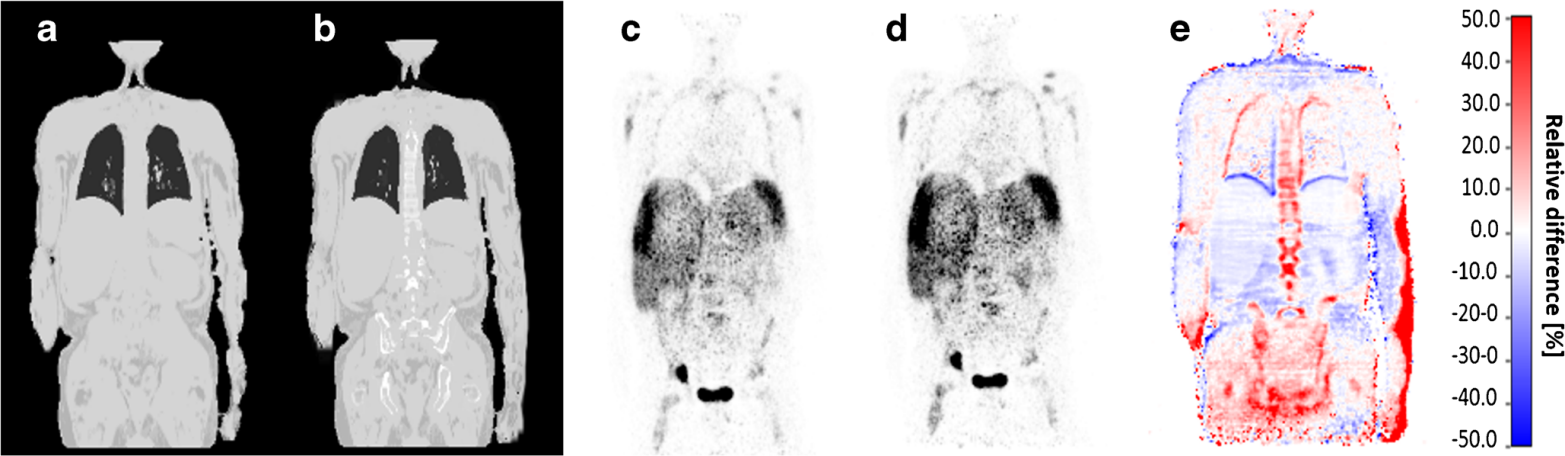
Patient example with injected ^68^Ga-DOTATOC: standard 4-compartment μ-map serving as reference (a) and improved 6-compartment μ-map (b) with corresponding attenuation corrected PET data (4-compartment (c), 6-compartment (d)). e A calculated relative PET difference map. Maximal relative differences between the 4- and 6-compartment AC PET data are observable in the truncated and bony areas also for this non-FDG tracer example
